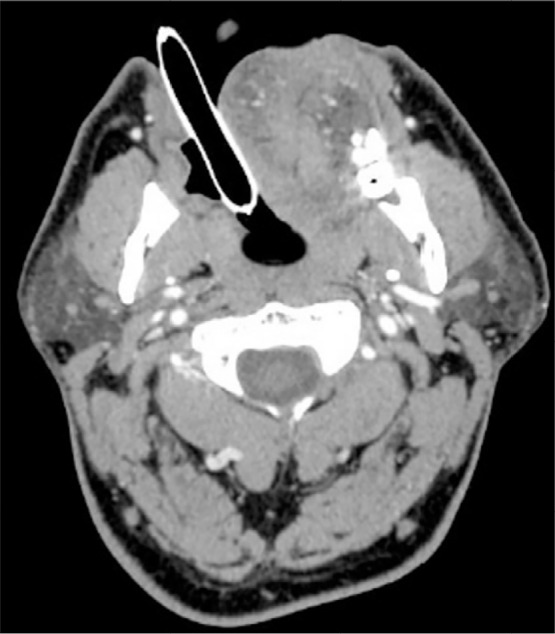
CT scan performed with patient intubated and assisted ventilation.
